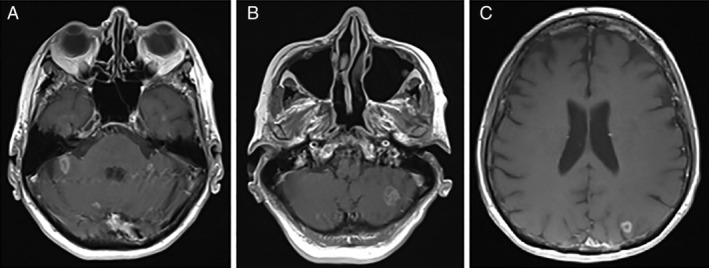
Axial T1‐weighted turbo‐spin echo (TSE) magnetic resonance imaging (MRI) demonstrating multifocal enhancing cerebellar (A, B) and cerebral lesions (C) with adjacent vasogenic oedema and no leptomeningeal involvement.
